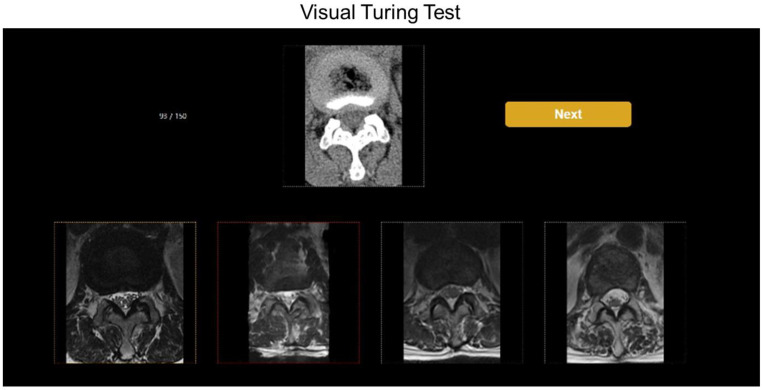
The validation set comprised 150 true and 450 synthetic images developed by 3 algorithms. The true and synthetic images were randomly mixed and displayed on the web solution. Four readers independently determined which MR image best reflected the axial CT image at the disc level and for real. The yellow and red frames of the MR images indicated the first and second choices, respectively. MR, magnetic resonance; CT, computed tomography.
